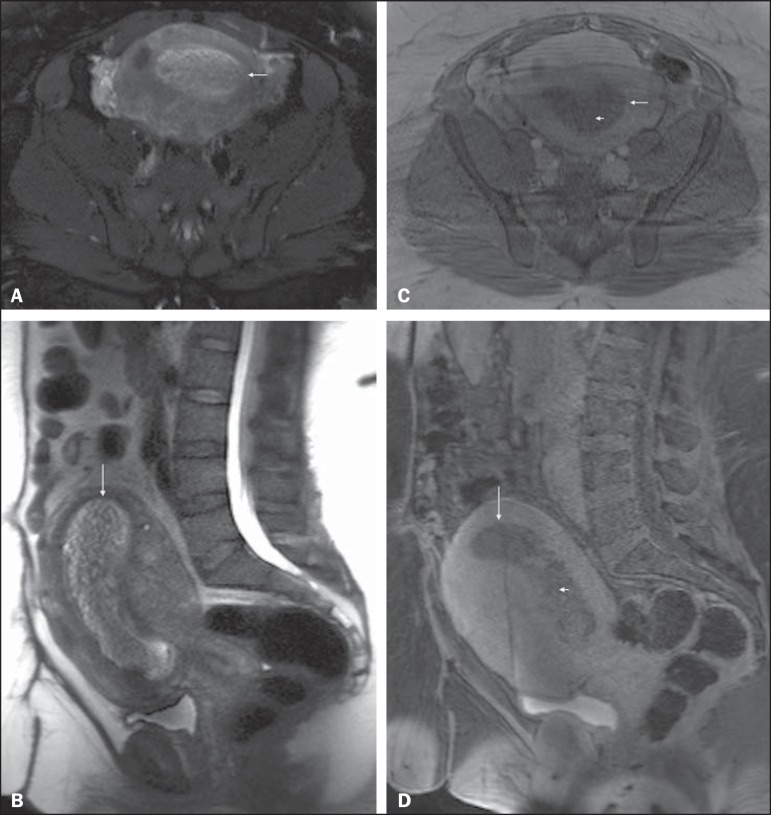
Pelvic MRI. Axial fat-suppressed (A) and sagittal
